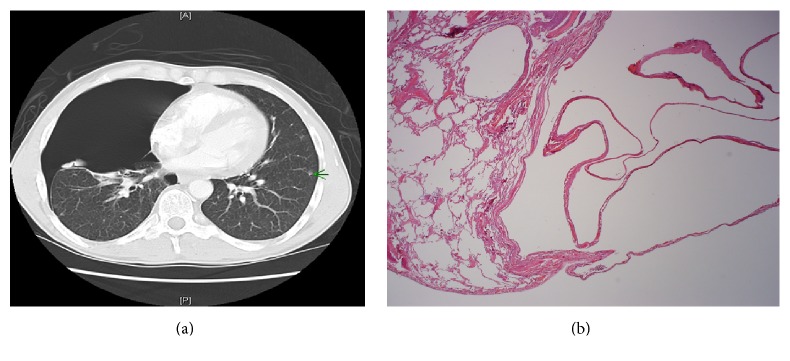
(a) HRCT of the lung showing pneumothorax on the right. Pneumothoraces tend to arise from the rupture of peripherally located cysts as pointed out on the left of the image (green arrow). (b) A low power view (×40) showing the ruptured cyst lined by a thin-walled alveolar epithelium.
